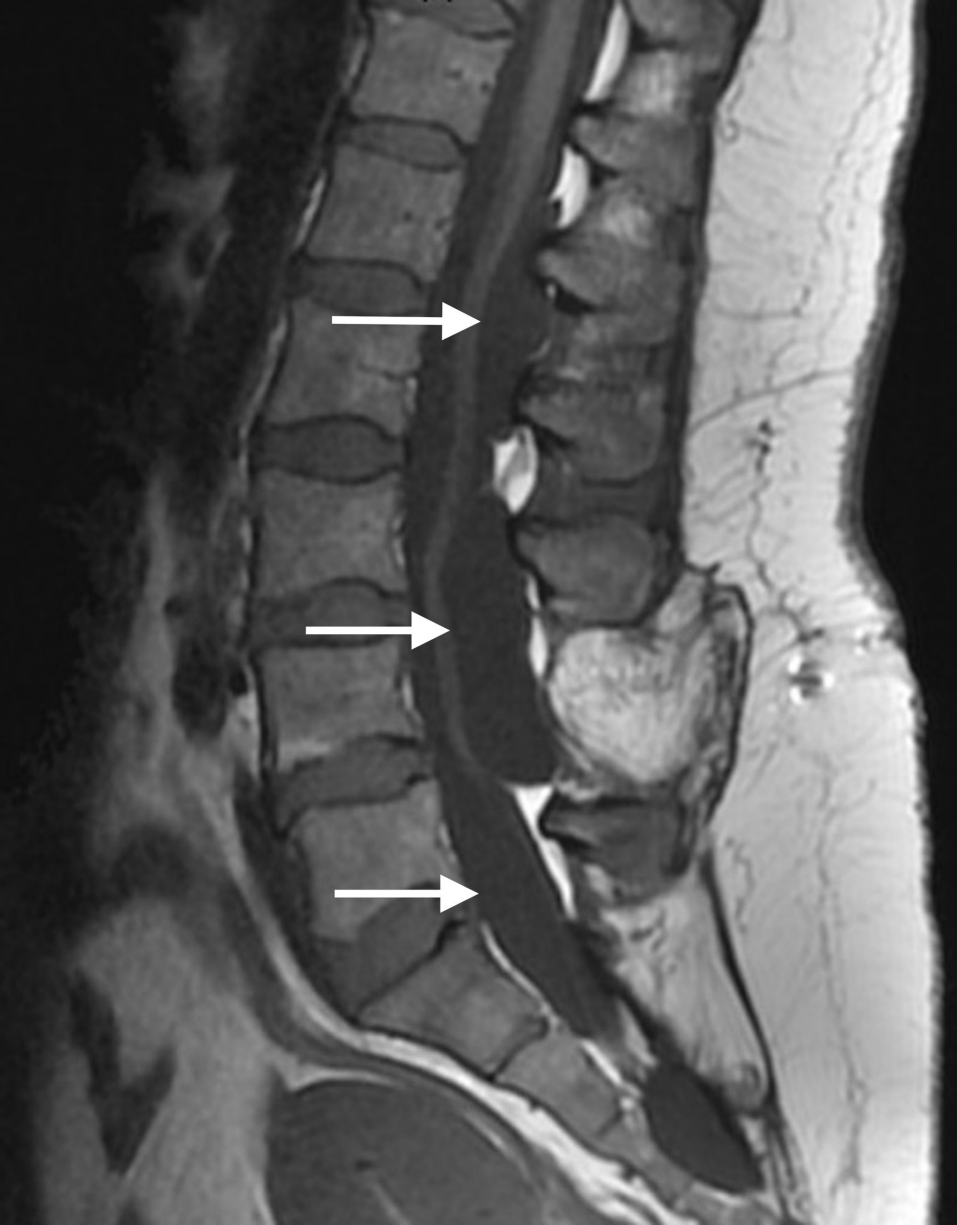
Sagittal T1 FSE MRI of the Spine FSE: fast spin echo; MRI: magnetic resonance imaging
